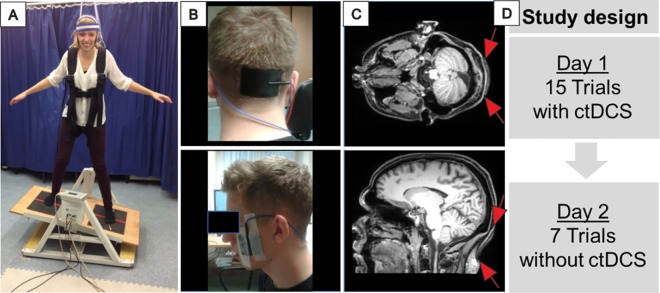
Experimental design. A: Subject performing the dynamic balance task on a Lafayette Instrument 16030 stability platform®. The individual shown in this figure has given written informed consent (as outlined in PLOS consent form) to publish these case details. Subjects were instructed to hold the platform in a horizontal position as long as possible, B: tDCS electrode position The top end of the cerebellar electrode (width 7 cm by height 5 cm) was centered 2 cm below the inion. Two reference electrodes were placed over the buccinator muscles bilaterally (5 cm x 5 cm). C: Position of the cerebellar electrode as revealed by axial and sagittal T1-weighted magnetic resonance imaging (MRI) scans in a healthy subject. Electrode paste leads to hyperintensive MRI signal. Position is indicated further by red arrows, D: Study design: Two days of training, cerebellar tDCS (ctDCS) was applied only on day 1. There were 15 trials on the first day and 7 trials on the second day of training, 30 seconds each.
